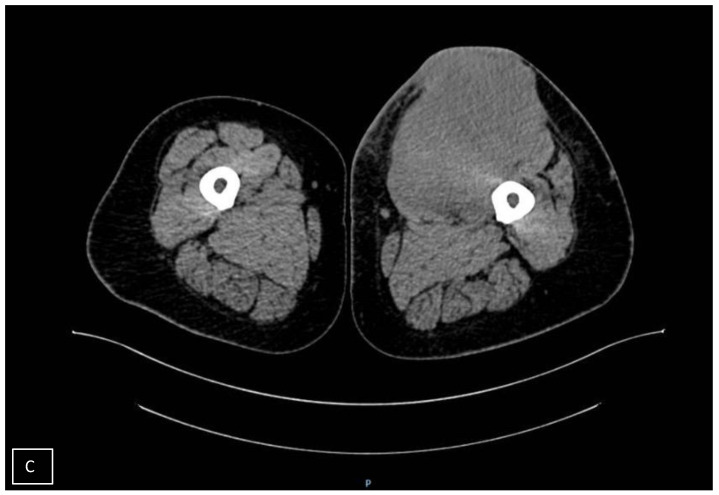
Nuclear magnetic resonance imaging (MRI) of the thighs. (A,B) Sagittal and coronal T1-weighted MRI. (C) Transverse T1-weighted MRI shows deep infiltration of the skin, the subcutaneous tissue, the left quadriceps, the vastus medialis, the vastus intermedius, and the rectus femoris muscles, and a highly intense irregular mass signal typical of the myxoid matrix in subcutaneous tissues, with an ill-defined margin in the upper third of the left thigh.
